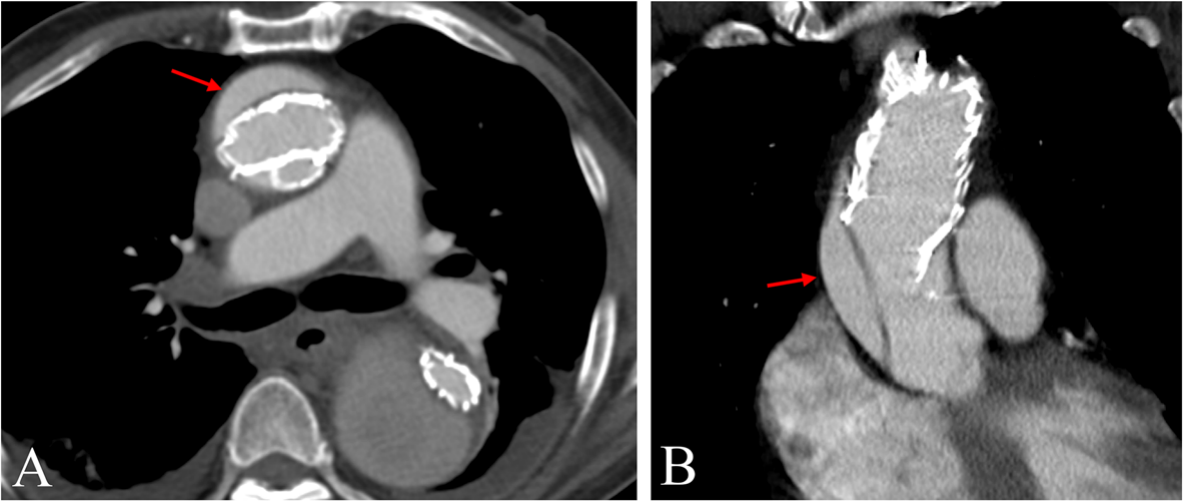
Follow-up enhancing chest CTA showed the migrating and new arising aortic dissection (red arrow) near proximal ascending aorta in axial (a) and coronal (b) view
